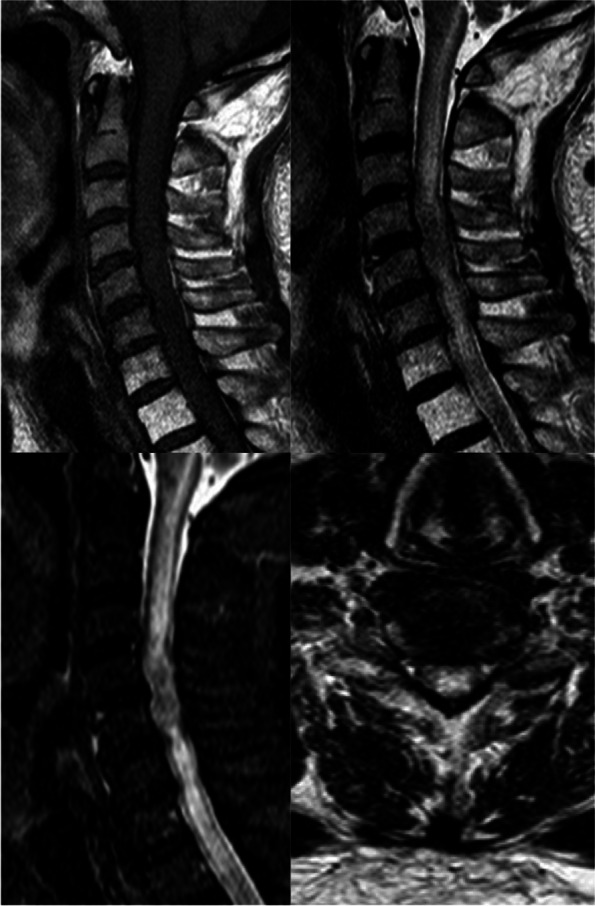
T2-weighted and STIR imaging demonstrated a hypo-isointense area in C4-5 with fusiform high intense of the surrounding spinal cord. The axial imaging suggested hyperintense intramedullary edema
